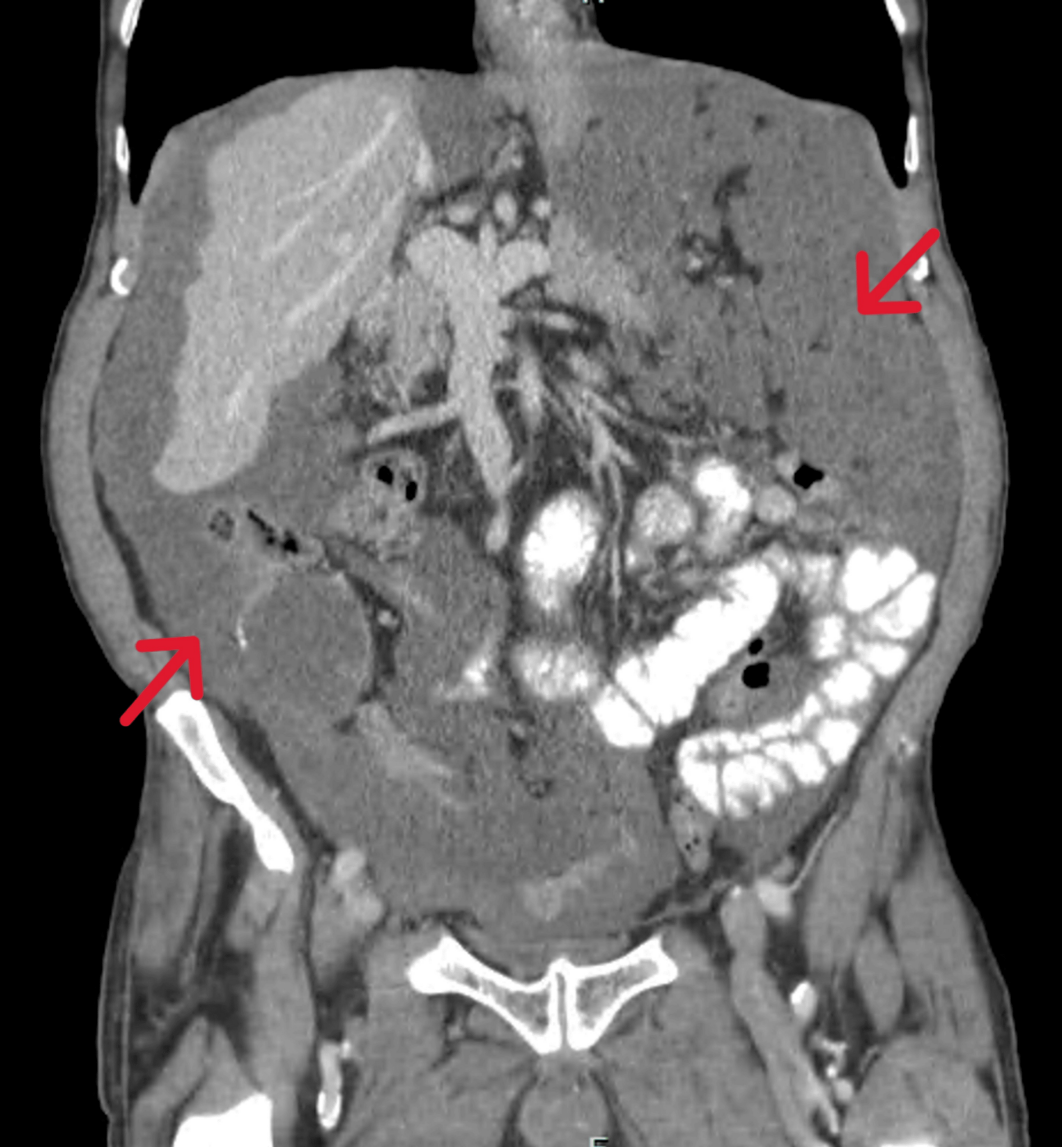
Abdominal CT (coronal view) The red arrows point at a mass compressing all the major intraperitoneal organs.
